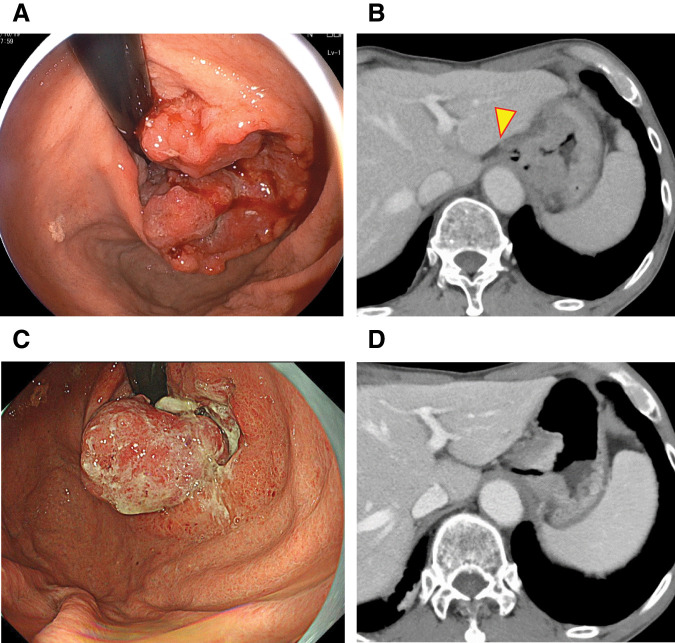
Imaging findings before and after preoperative chemotherapy (A) Upper gastrointestinal endoscopy before preoperative chemotherapy showing a type 2 tumor at the esophagogastric junction. (B) Contrast-enhanced CT before preoperative chemotherapy showing wall thickening mainly at the esophagogastric junction and (the yellow arrowhead) local partial invasion of the left diaphragmatic crus. (C) Upper gastrointestinal endoscopy after preoperative chemotherapy showing a slight reduction in tumor size. (D) Contrast-enhanced CT after preoperative chemotherapy showing slight improvement in the tumor size and wall thickening, with no distant metastases or new lesions.
